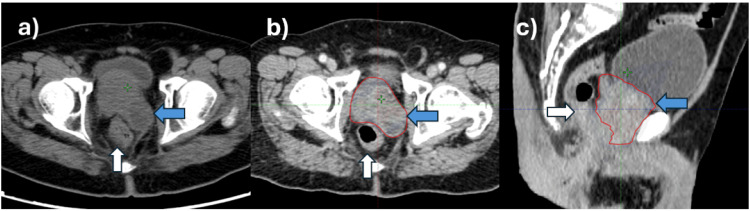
CT image (a) axial section shows a tumor in the prostate with a cystic component (blue arrow) on the left side impinging on the rectum (white arrow), (b) target volume (blue arrow) encompassing the entire prostate and cystic component on the left side in the axial section, and (c) sagittal section CT: computed tomography
